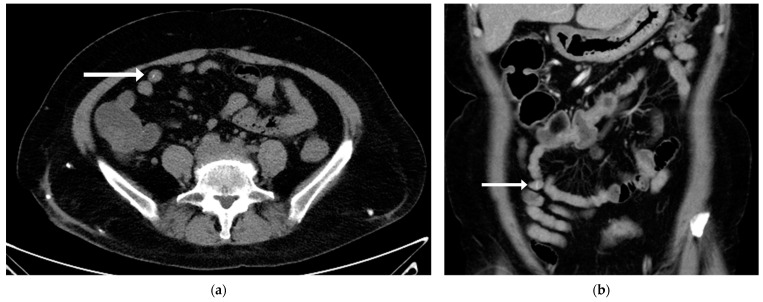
The abdominal computed tomography (CT) revealed a linear hyperdense content (fish bone) within the terminal ileum. (a) The axial view of the non-contrast abdominal CT showed a continuous hyperdense spot within the ileum, indicated by the arrow. (b) The coronal view of the contrast-enhanced abdominal CT demonstrated a linear hyperdense object consistent with the axial view, indicated by the arrow.
